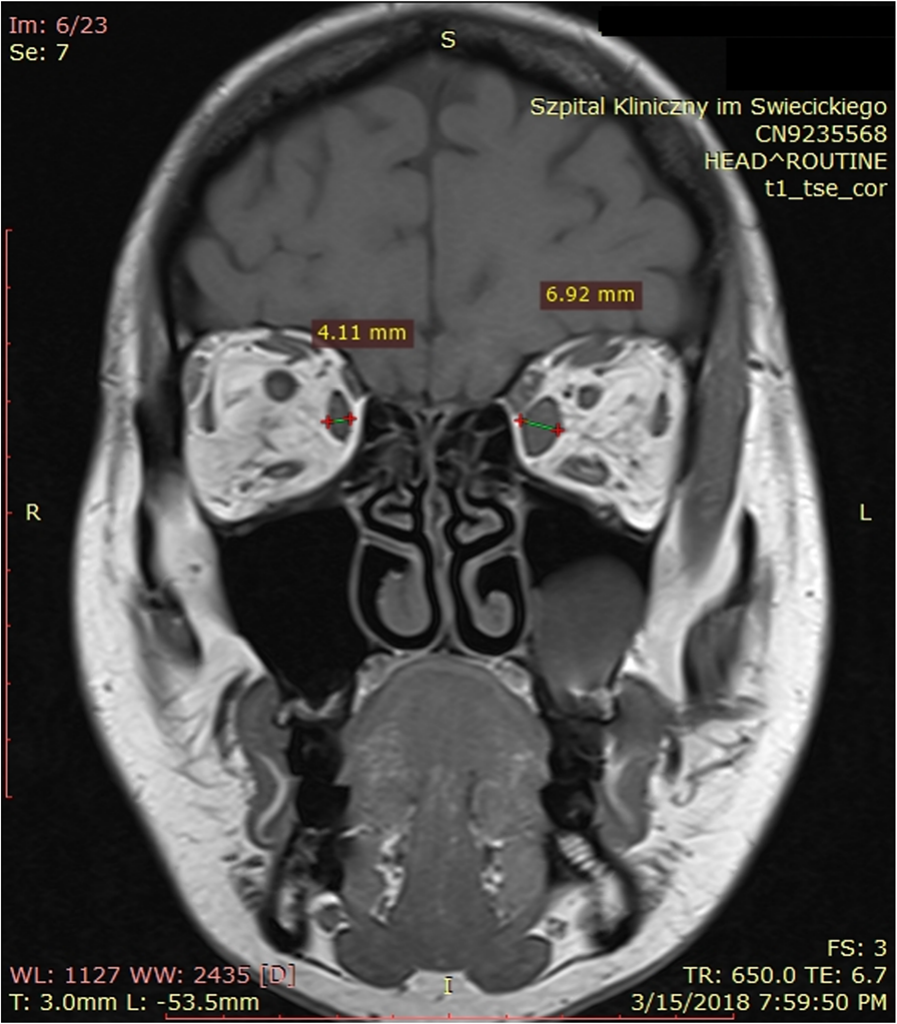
Thickening of the left medial rectus muscle demonstrated by MRI in a coronal plane. In addition, slight thickening of the inferior rectus muscle is also visible. Besides, a large cyst in the left maxillary sinus is visible sized 23 mm
